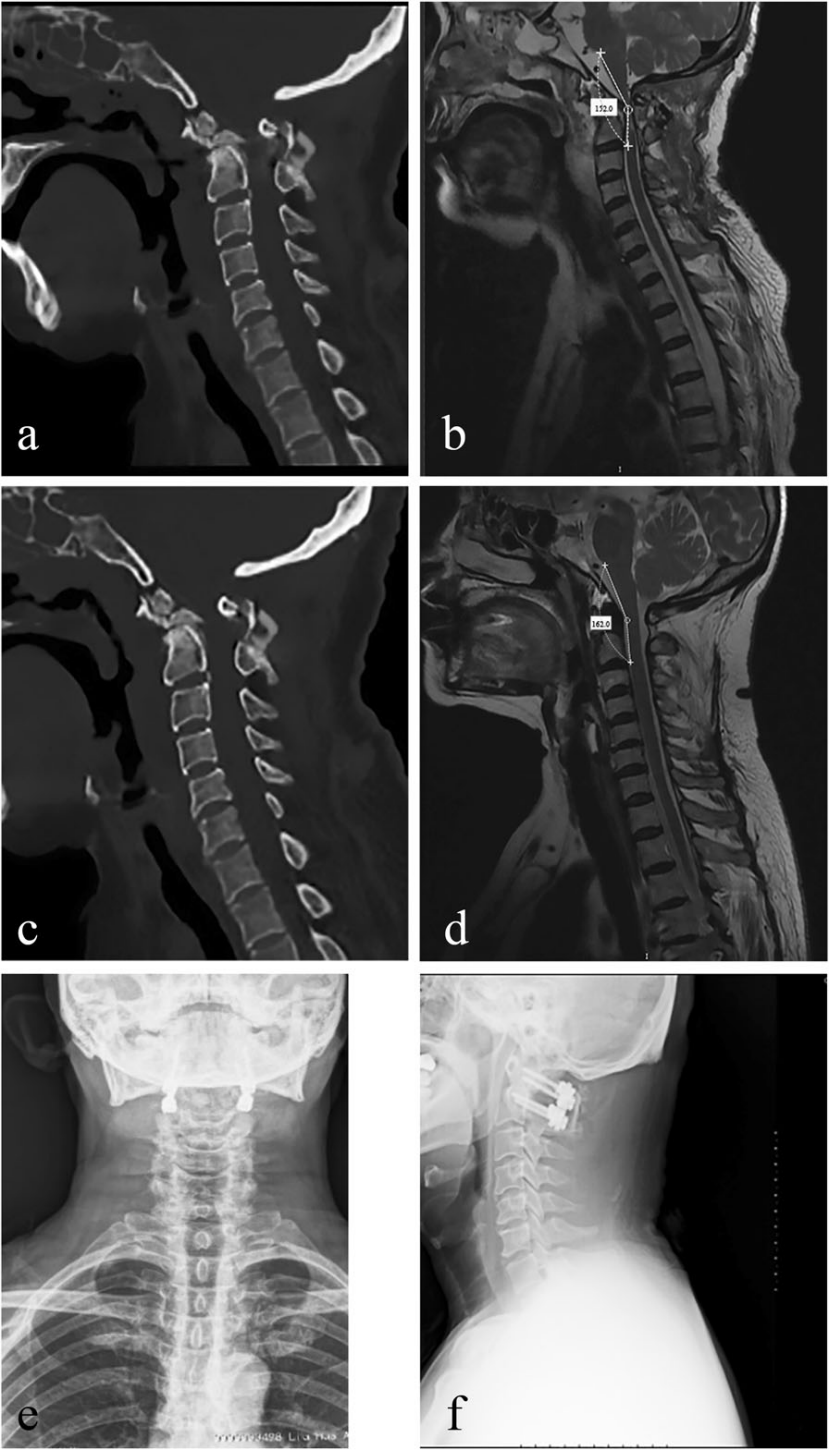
a Sagittal CT showed reduction and restoration 1 month after operation. b MRI sagittal T2-weighted films 1 month after operation showed ventral medullary decompression with CMA recovery to approximately 152.00°. c At 13 months follow-up, sagittal CT showed no instrument loosening, no loss of reduction, and evidence of bony fusion. d At 13 months follow-up, MRI sagittal T2-weighted image showed CMA recovery to approximately 162.00°. e, f Postoperative X-ray showed good internal fixation
